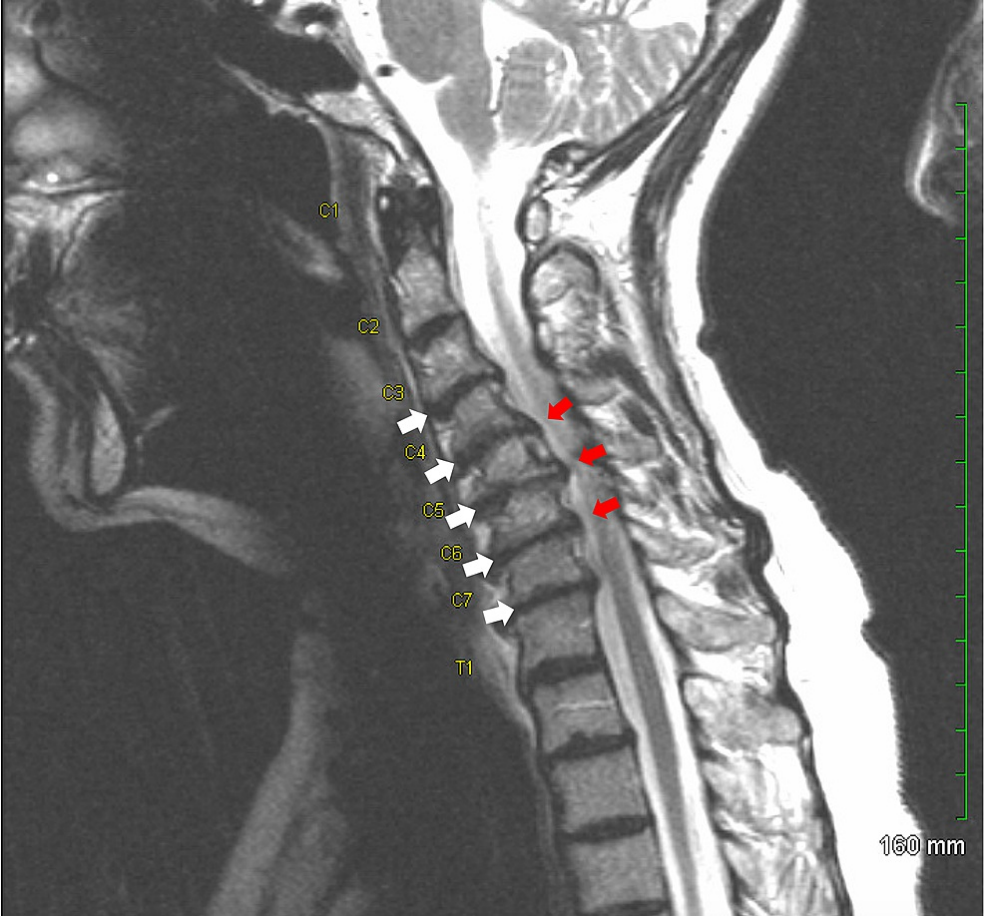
Sagittal view MRI of the cervical spine Multilevel cervical degenerative disc disease with loss of normal cervical lordosis (white arrows) Levels of mild to severe central stenosis from C4 to T1 (red arrows)
